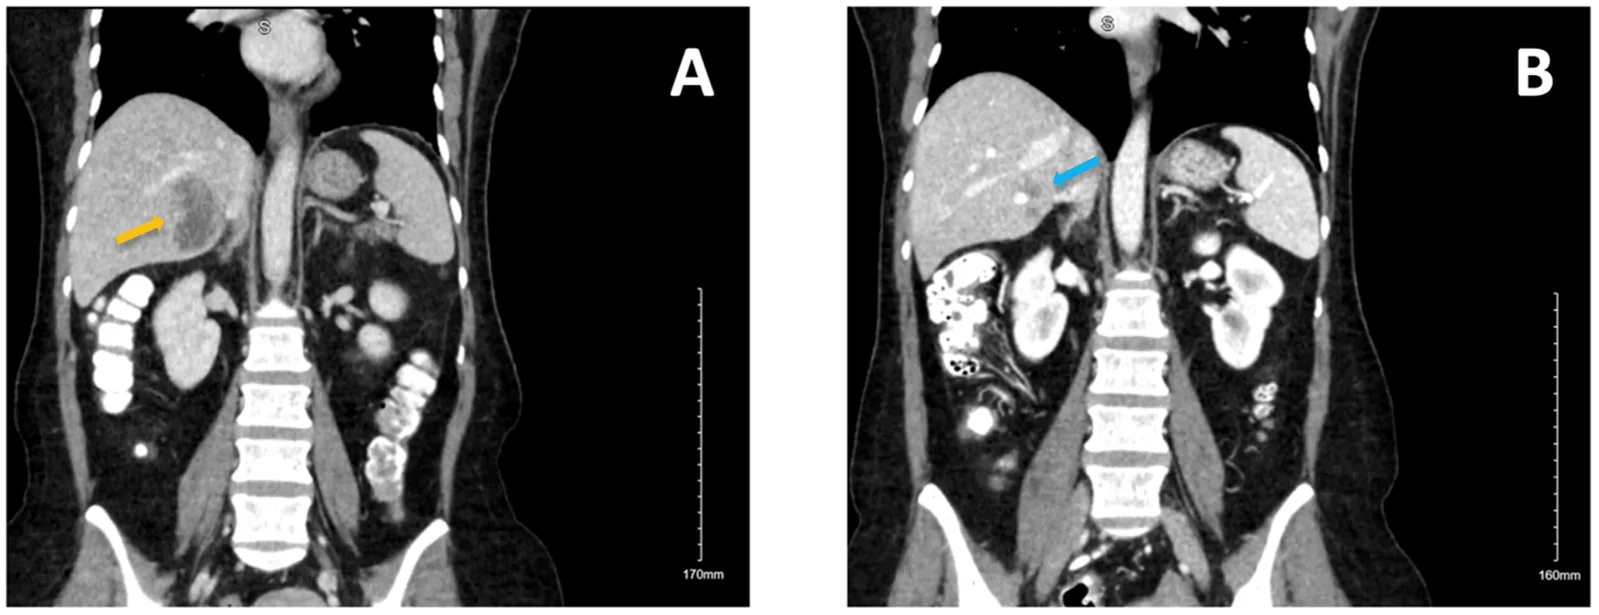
Coronal CT views of a complex, heterogenous lesion (yellow arrow) in the right hepatic lobe, initially measuring 5.2 x 2.8 x 4.2 cm (A). Interval decreases in size (blue arrow) are noted on subsequent imaging, down to 2.2 x 2.2 x 1.2 cm (B).
